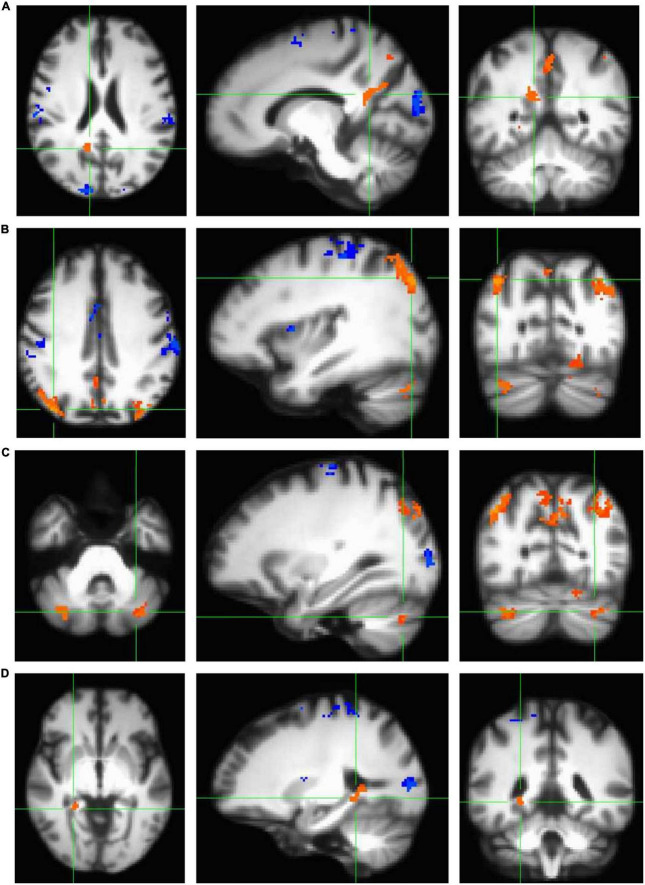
Clusters that demonstrated statistically significant activation (*p* < 0.001) during the navigation task in encoding > control conditions (orange) and control > encoding conditions (blue) as measured using a standard univariate analysis in AFNI (*3dttest*++ with ETAC). All clusters shown here survived correction for multiple comparisons and are demonstrated on the age-specific MRI brain template warped to MNI space. Note that all four panels (A–D) show a significant encoding > control cluster in three planes, listed left to right: axial (left = right hemisphere), sagittal (left = anterior), and coronal (left = right hemisphere). (A) Significant cluster comprising the bilateral retrosplenial cortex and right precuneus. (B) Two significant clusters comprising bilateral inferior parietal cortex. (C) Two significant clusters comprising the bilateral cerebellum (lobule VIIa Crus I and II). (D) Significant cluster comprising the medial temporal areas, including right fusiform gyrus, right parahippocampal gyrus (and more specifically, parahippocampal cortex), right lingual gyrus, and right hippocampus.
